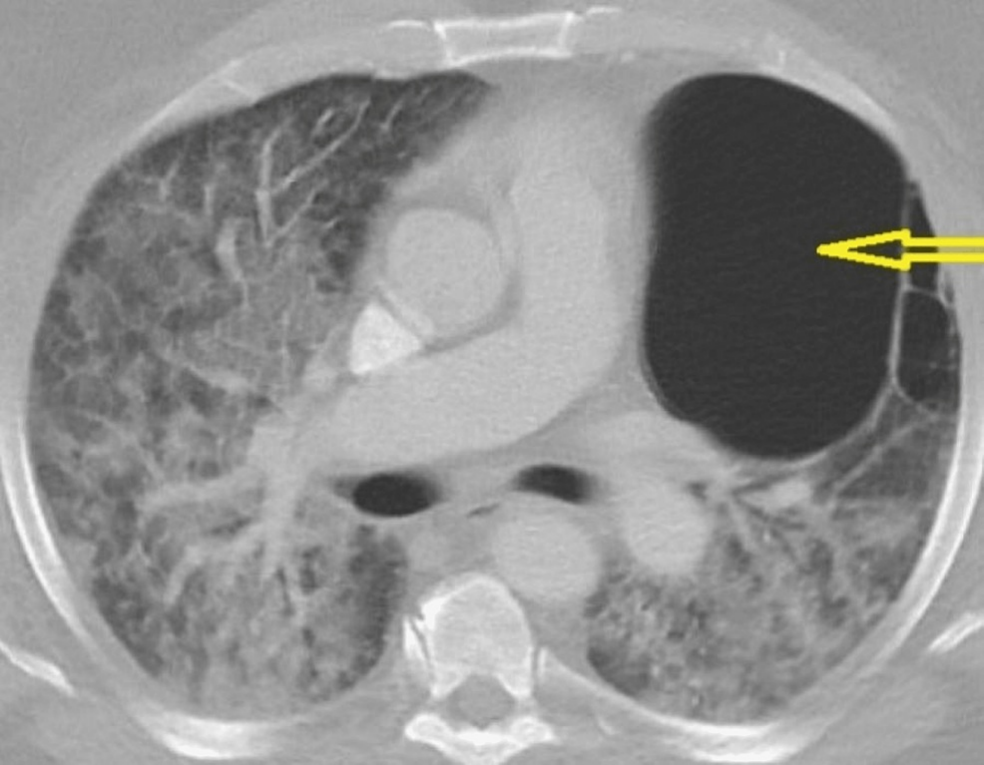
Computed tomographic angiogram. No pulmonary embolism was noted. A 13-cm bulla was noted in the left lung (yellow arrow) with bilaterally ground-glass consolidation. Differential diagnosis includes pulmonary edema, pneumonia, or various causes of alveolitis.
